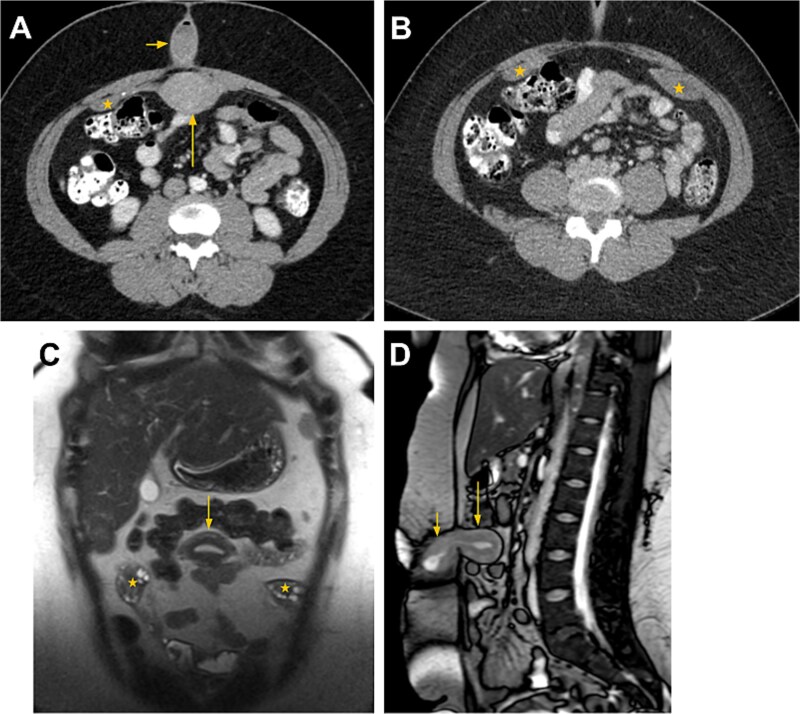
CT and MRI findings after uterine transposition. (A, B) Axial CT images of the abdomen and pelvis at the level of the umbilicus following intravenous and oral iodinated contrast administration show the transposed uterus (long arrow in A) located in the anterior midline abdomen and the neocervical umbilical canal containing a small amount of fluid and minimal air (short arrow in A). The bilateral ovaries have also been transposed and appear as intraperitoneal, featureless structures along the anterior abdominal wall (* in A and B). (C) Coronal T2-weighted MRI image demonstrating the intraabdominal, intraperitoneal position of the uterus (arrow in C) and bilateral ovaries (* in C). The characteristic uterine zonal anatomy-outer myometrium with intermediate T2 signal, junctional zone with low T2 signal, and endometrium with high T2 signal-helps differentiate the uterus from adjacent bowel loops. Physiologic ovarian cysts, when present, assist in identifying the intraabdominal ovaries. (D) Sagittal gradient-echo MRI image showing the intraabdominal, intraperitoneal uterus (long arrow in D) and the cervix (short arrow in D) within the umbilical canal.
